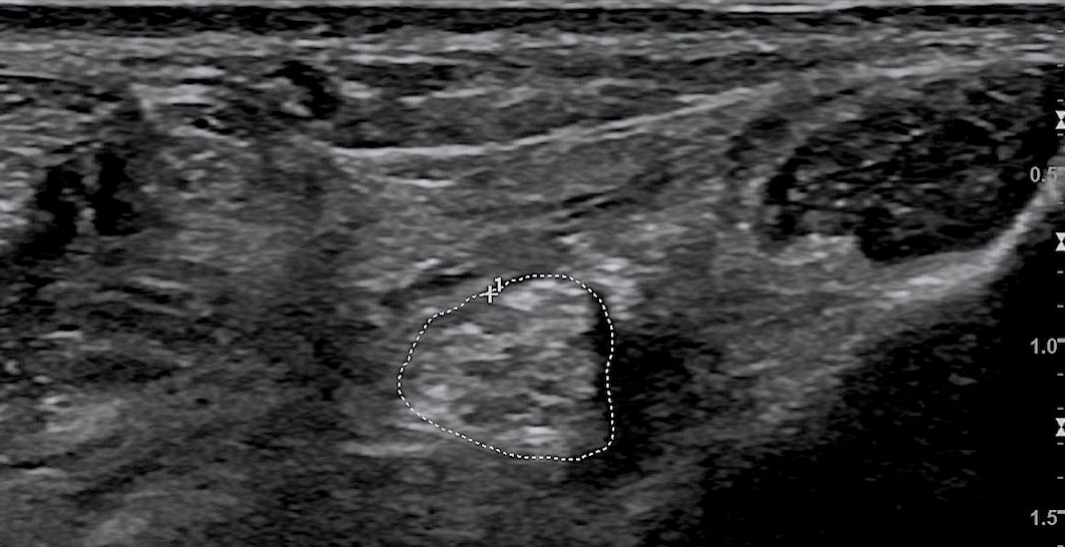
Color Doppler ultrasound image of the tibial nerve. The area circled by the dashed line is the CSA of the tibial nerve. CSA, cross-sectional area.
